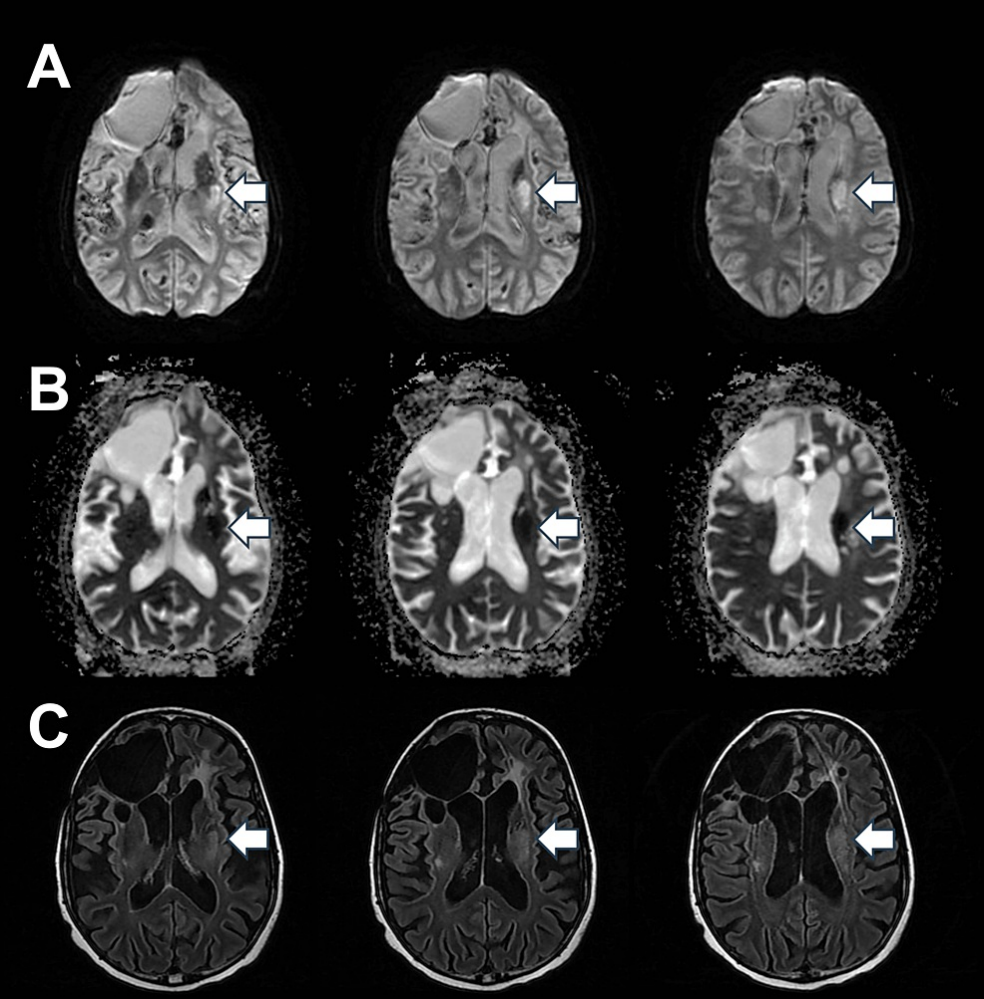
Brain MRI with (A) diffusion-weighted imaging, (B) apparent diffusion coefficient, and (C) fluid-attenuated inversion recovery sequences showing acute left corona radiata/caudate body stroke extending to the external capsule (arrows)
